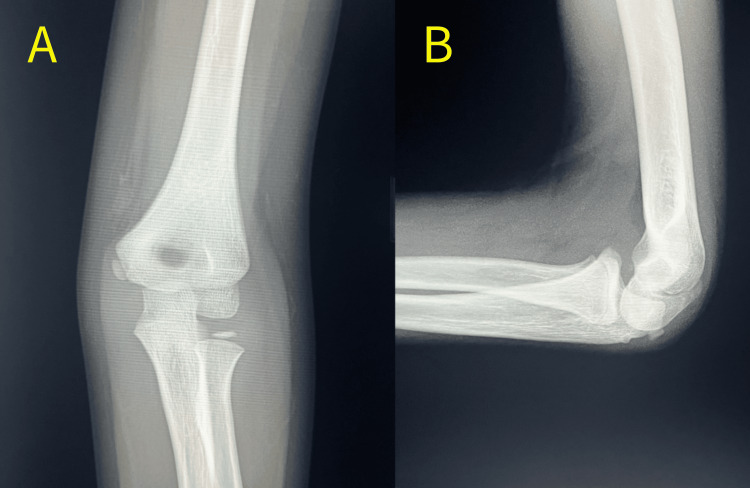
X-ray of anteroposterior (A) and lateral (B) views of the elbow joint six weeks after the initial injury. Bone healing without any deformation was obtained.
